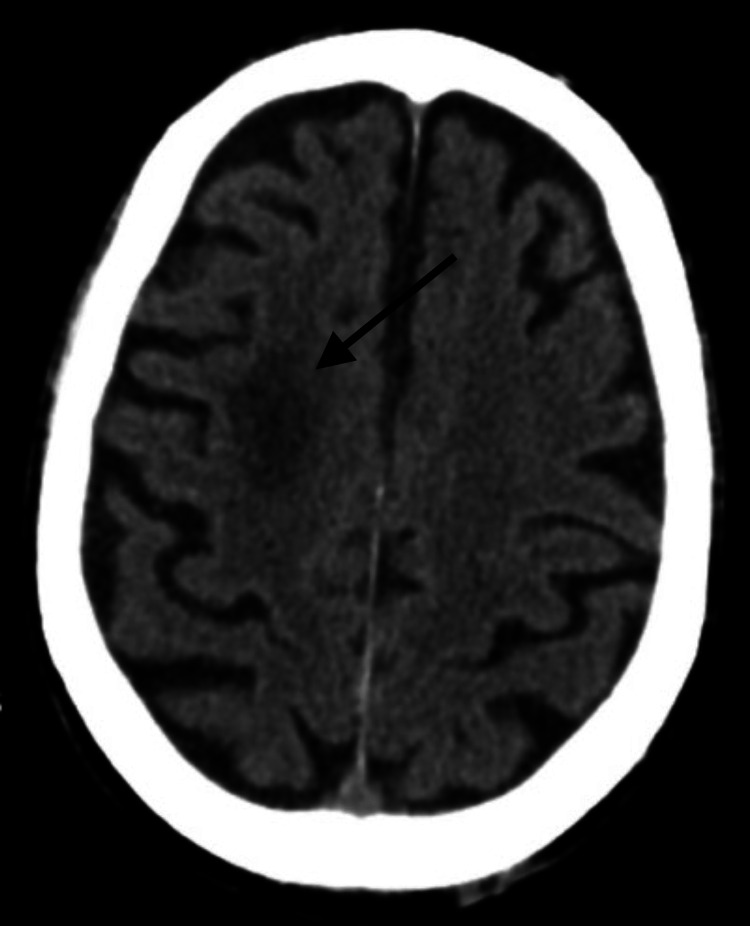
Head CT showing ischemic lesion sequelae.
